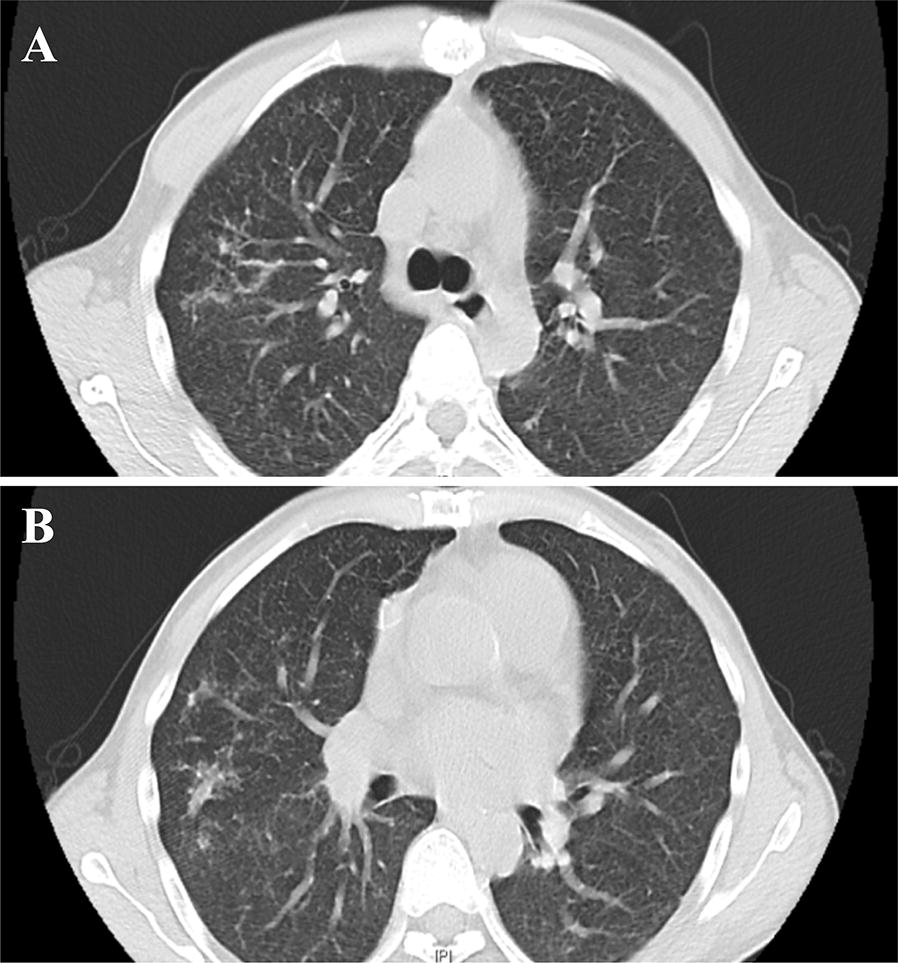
Axial computed tomography (CT) scan of the chest showing thickened lung markings with patchy shadows in both the upper lobes (A) and the lower lobes (B)
